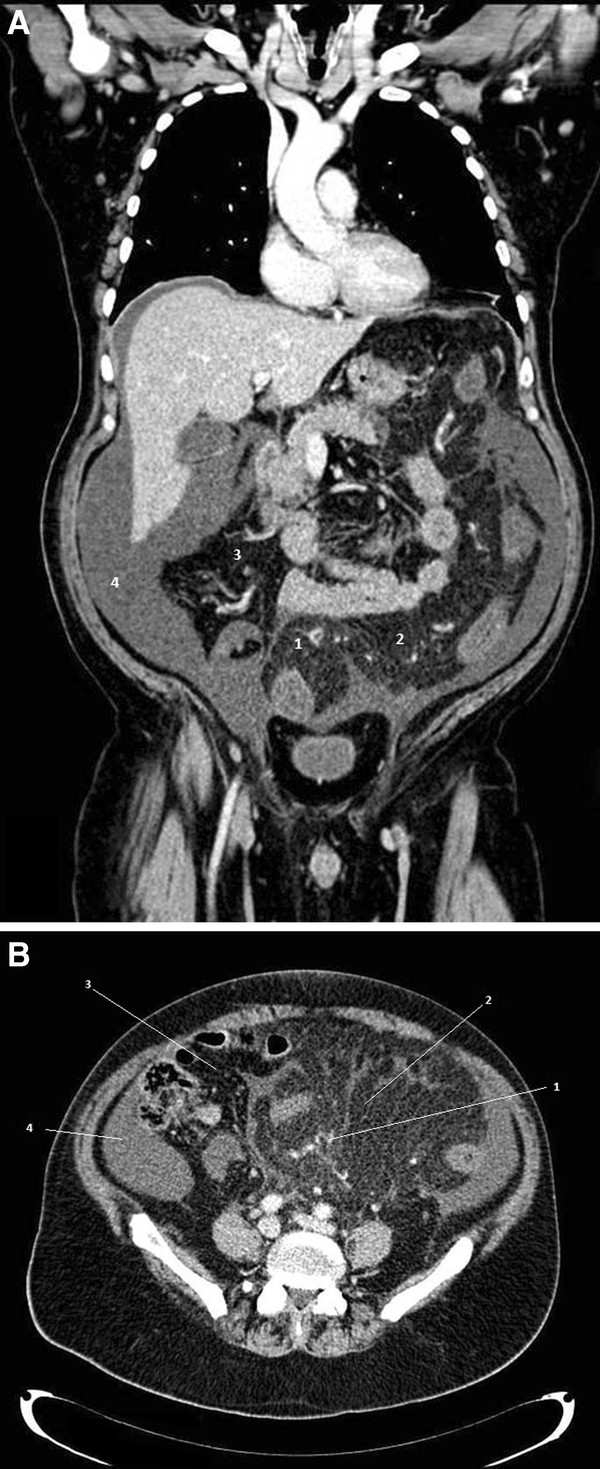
A and B: CT-scan showing mesocolic panniculitis. Contrast-enhanced coronal (1A) and axial (1B) CT scan showing mesocolic enlargement with hypervascularization (1), increased density of the mesocolic fat (2) compared with mesenteric fat (3) and ascites (4) suggestive of mesocolic panniculitis of the sigmoid and the ascending colon.
